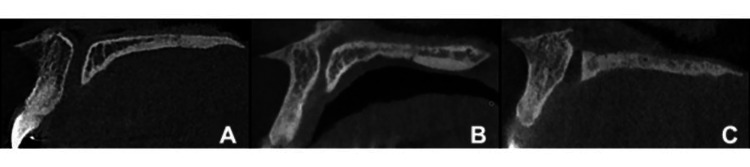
CBCT-images showing the three shapes of the nasopalatine canal A: Cylindrical shape (type A); B: A canal with a branch in the upper part (type B); C: A canal with a branch in the middle part (type C) CBCT: Cone beam computed tomography
